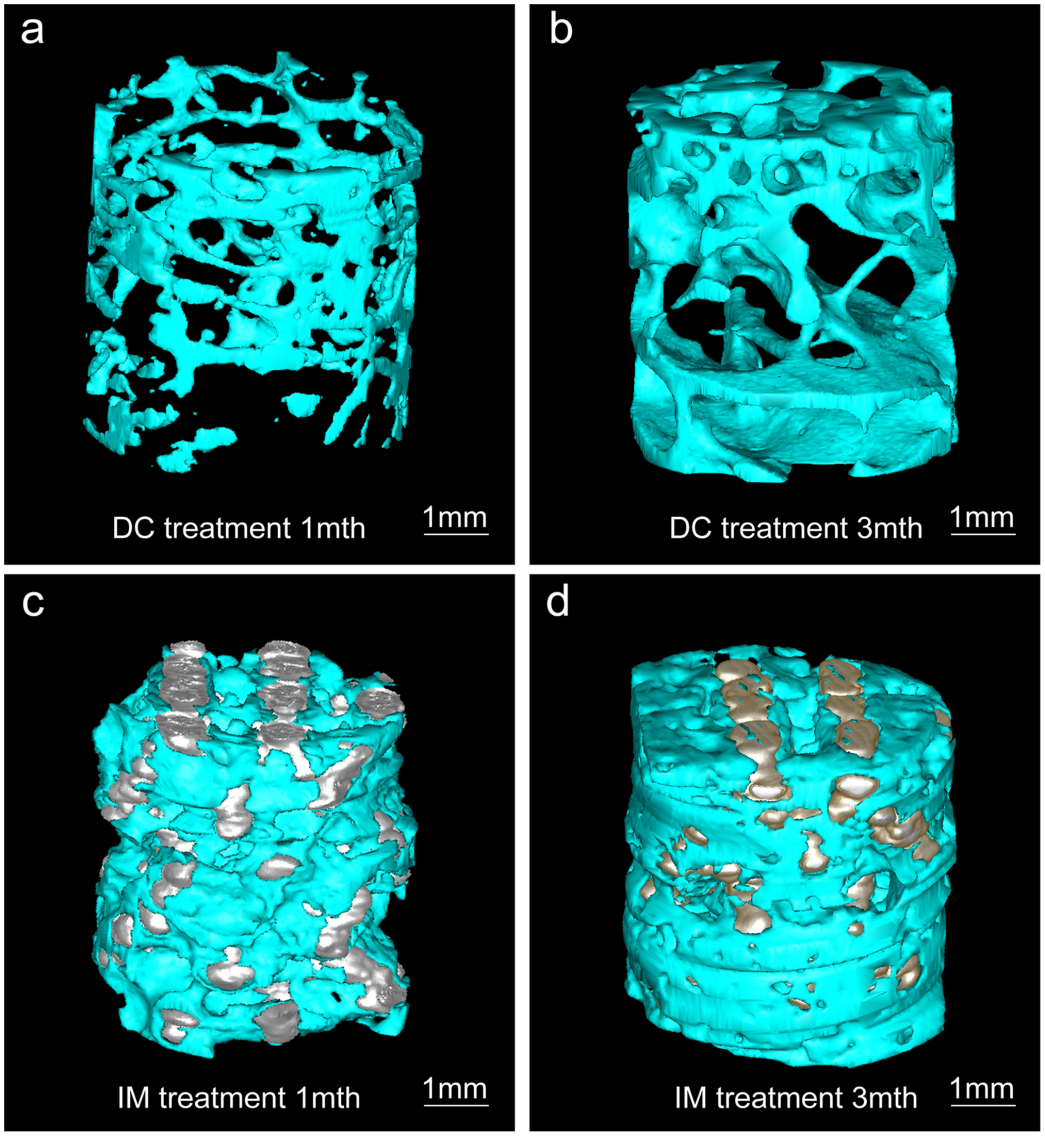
Lateral radiographic images of the ankle at different times. White arrowheads indicate osteolytic changes inside the talus, and white triangles indicate titanium rods.
